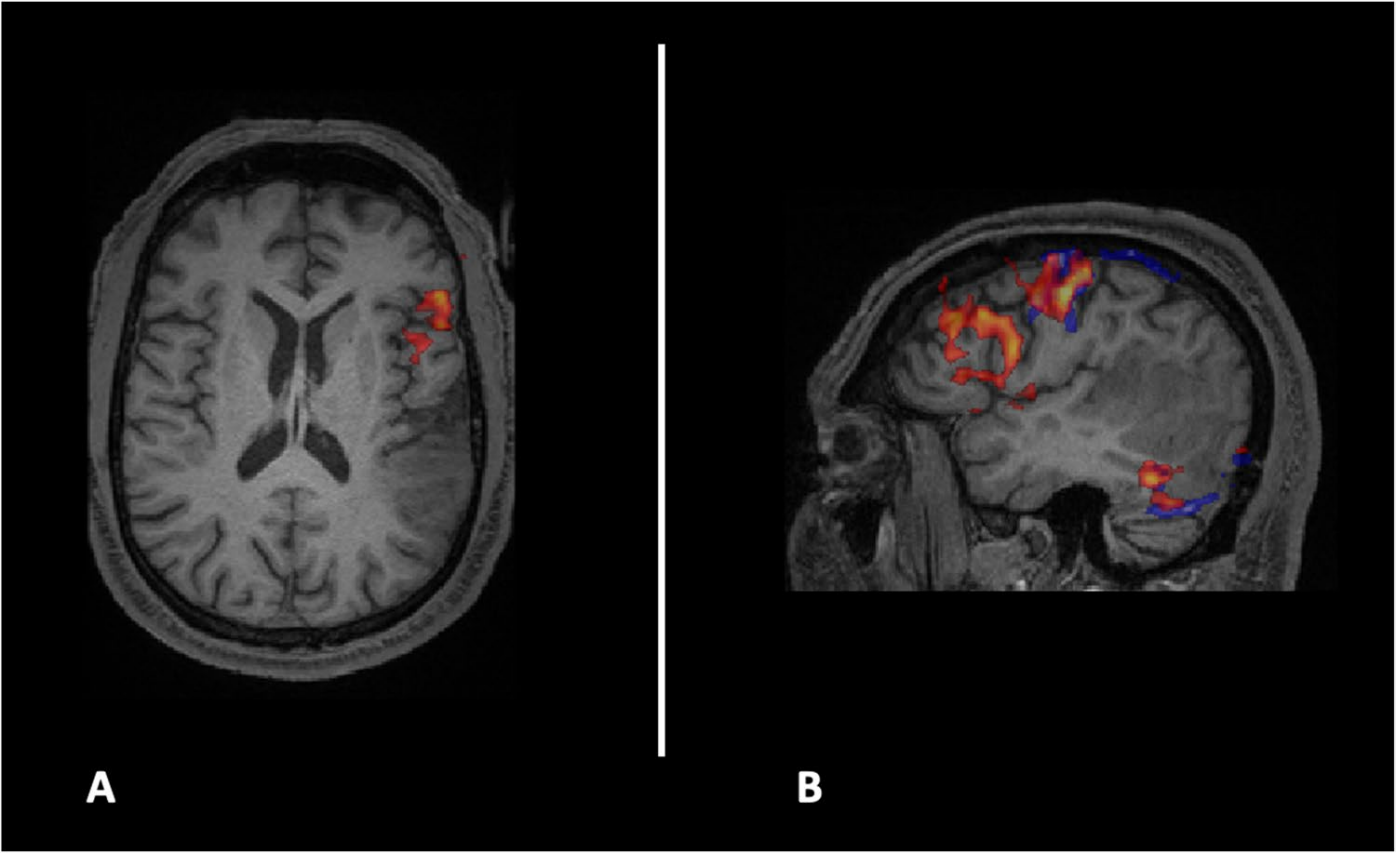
Functional magnetic resonance imaging showing (A, axial view; B: sagittal view) lateralization of the language to the left hemisphere, with activations near the lesion in the left temporal posterior region. Red corresponds to the sentence completion task; blue corresponds to the antonym generation task
